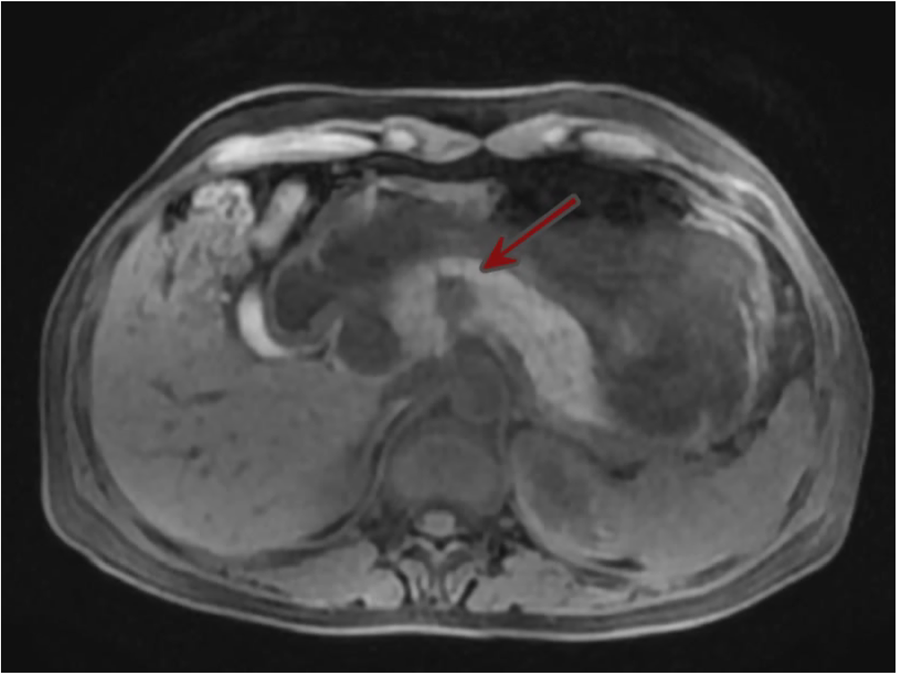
MRI disclosed a mass in the pancreatic neck.
